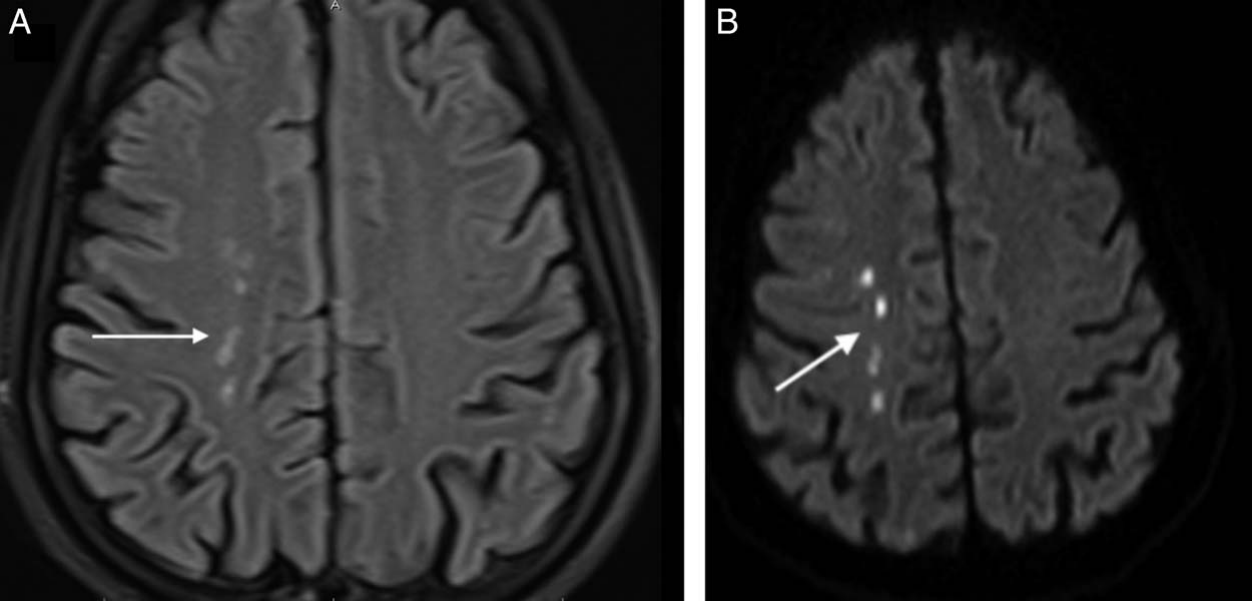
A 55-year-old COVID-19 positive male presenting with ptosis. (A) FLAIR axial image showing multiple flair high signal intensity (white arrow) in watershed region of right anterior cerebral and middle cerebral artery. (B) DWI image of the same patient shows restriction of these lesions (white arrow) suggestive of multifocal infarct.
